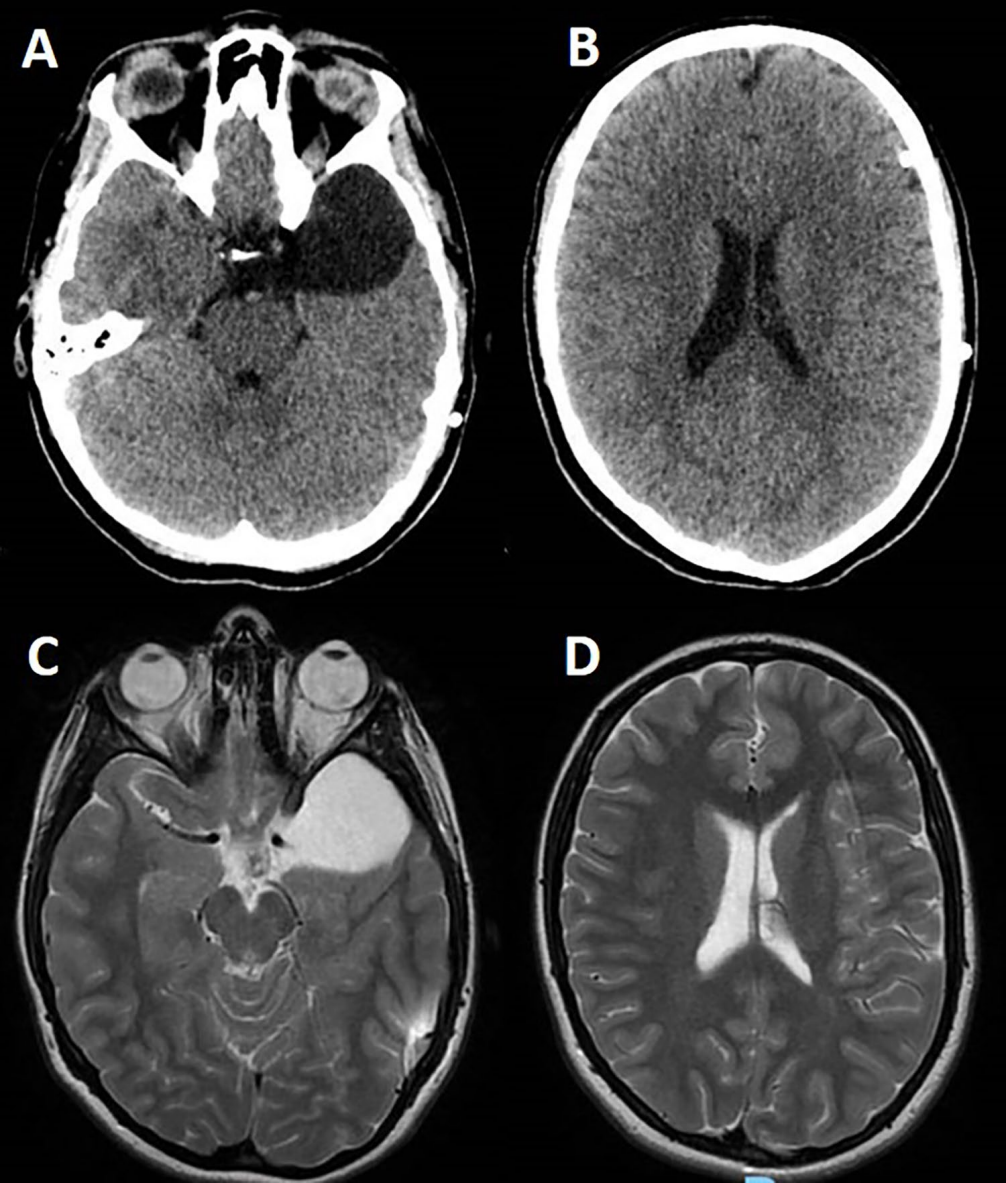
CT scan (A, B) and MRI (C, D) showing the complete resolution of the hygroma. The cyst is unchanged
